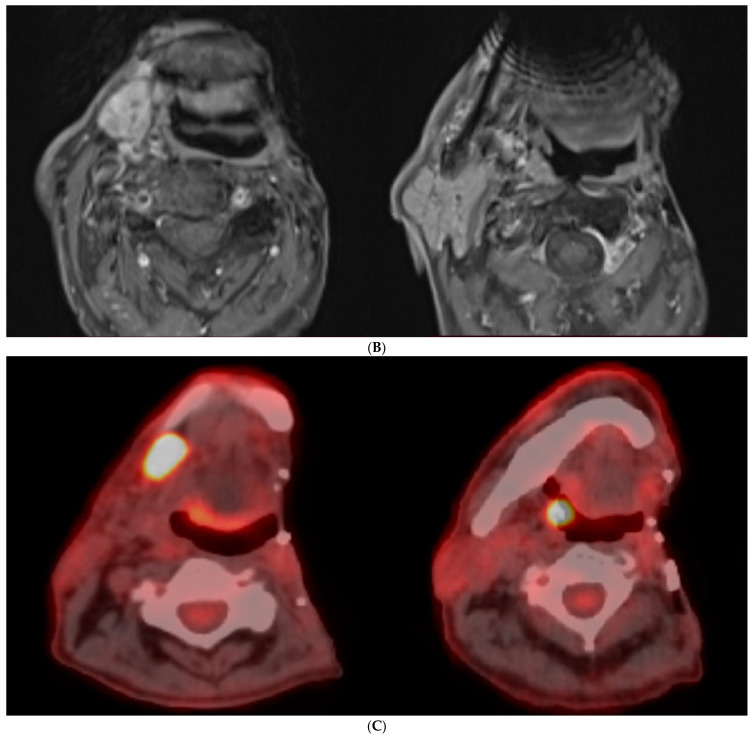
(A) CT, (B) MRI, and (C) PET-CT of the second primary disease on the contralateral neck. (A: anterior, P: posterior, R: right, L: left, S: superior.) The avidity in the image 2C depicts the two areas where the disease is evident in the PET-CT.
